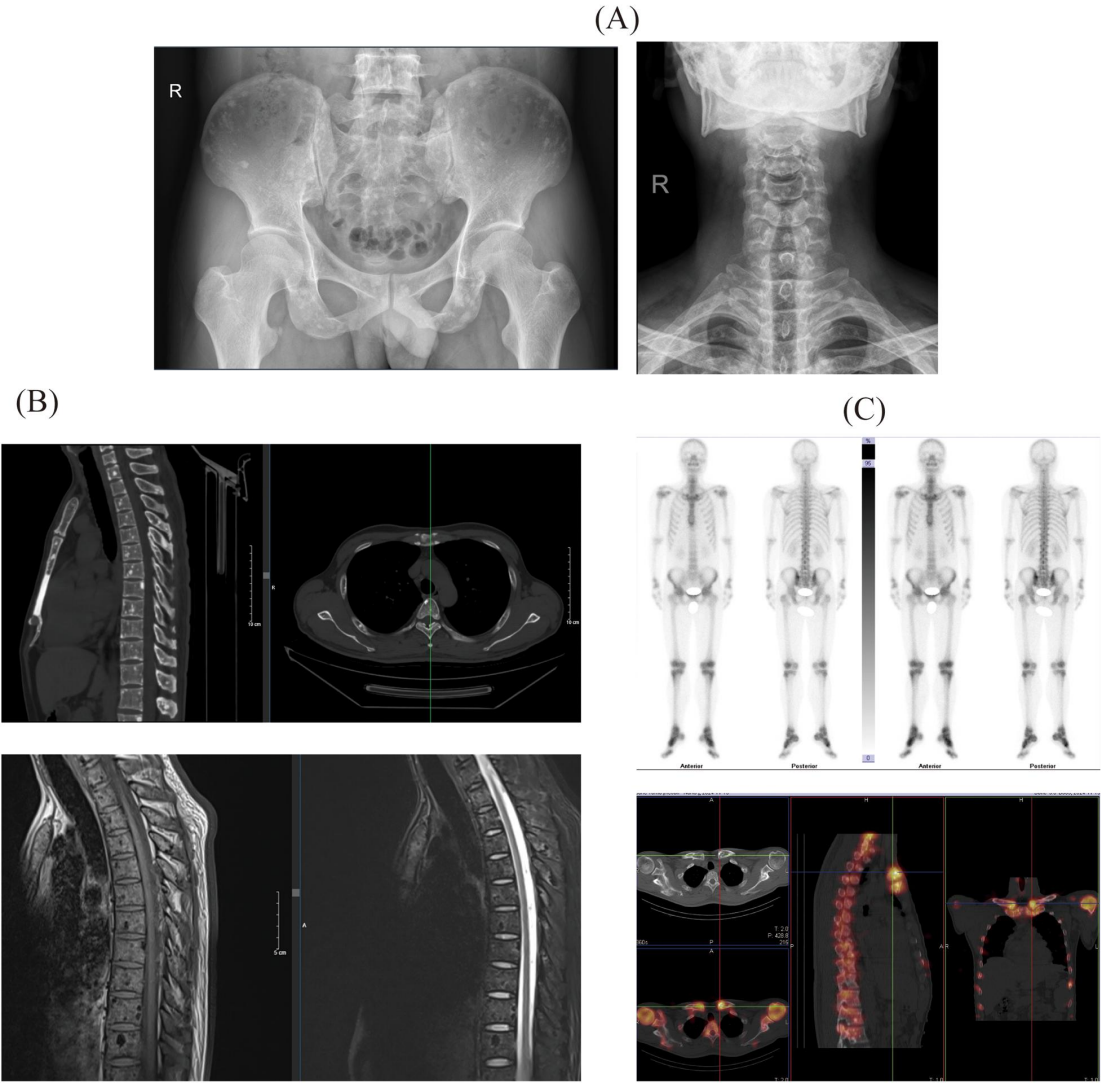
The results of the patient's whole-body imaging examination include: (A) x-ray images of the pelvis and cervical spine; (B) computed tomography (CT) and magnetic resonance imaging (MRI) results of the spine; (C) single-photon emission computed tomography (SPECT) results of the patient.
